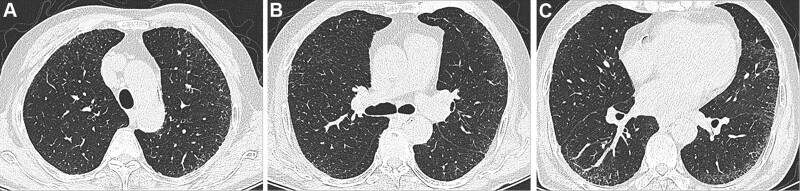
Representative CT images at different levels of the same volunteer. M, 70 yr—ILST, ILIT, and RS of bilateral subpleural area. (A) Upper lung level; (B) Middle lung level; (C) Lower lung level. CT = computed tomography, ILIT = intralobular interstitial thickening, ILST = interlobular septal thickening, RS = reticular shadow.
